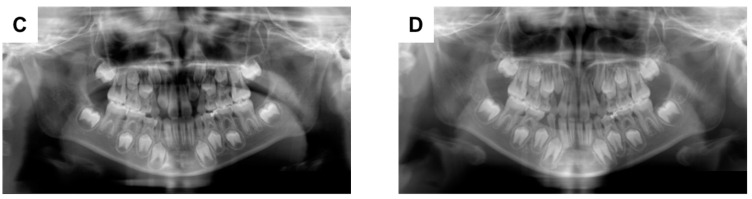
Orthopantomographs taken during follow-up. When the patient was aged (A) 5 years and 10 months; (B) 8 years and 3 months; (C) 9 years; and (D) 10 years and 1 month.
